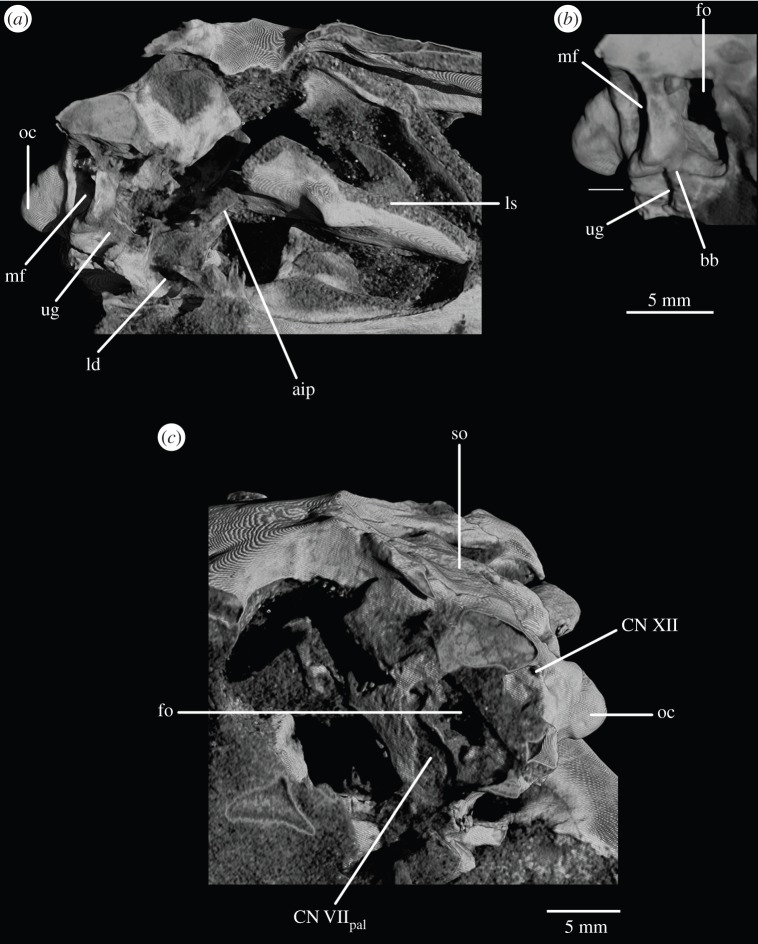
CT reconstructions of braincase of SAM-PK-5867 in (*a*) right lateral view (cross section through skull), (*b*) right lateral view showing the ‘bridge of bone’ (basisphenoid–opisthotic contact) and (*c*) left lateral view (cross section through skull). For abbreviations, see table 1.
